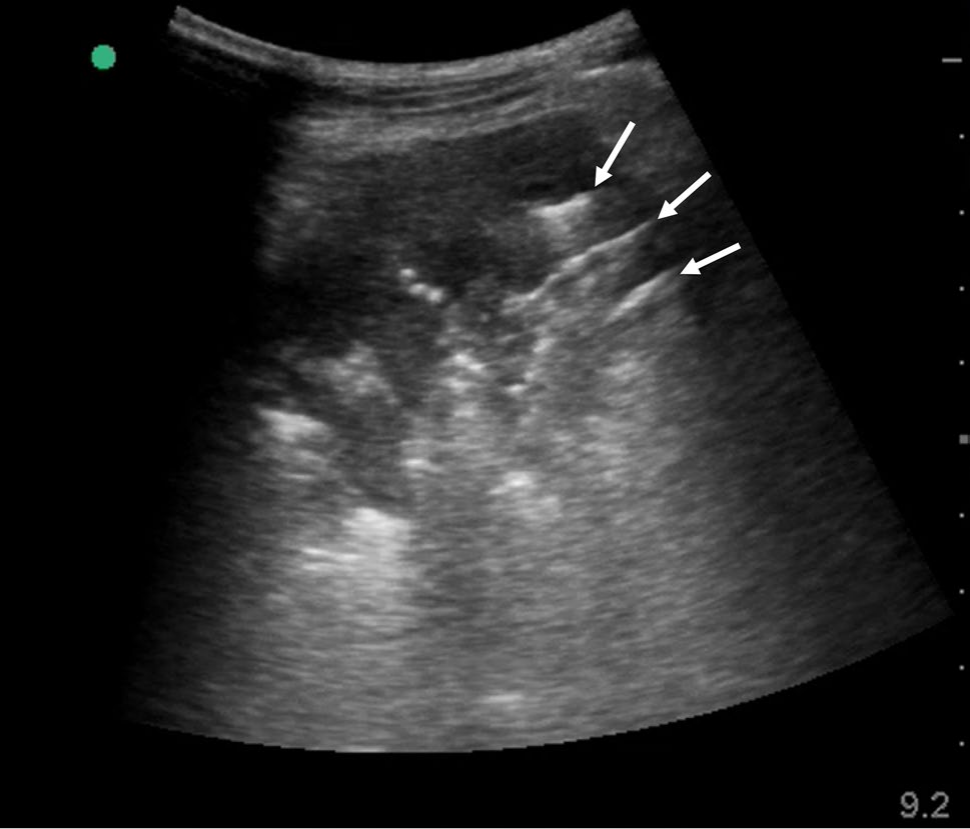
A lung ultrasound image of community-acquired pneumonia. A large lobar consolidation with irregular and serrated margins; the area of consolidation includes sonographic air bronchogram (arrows)
